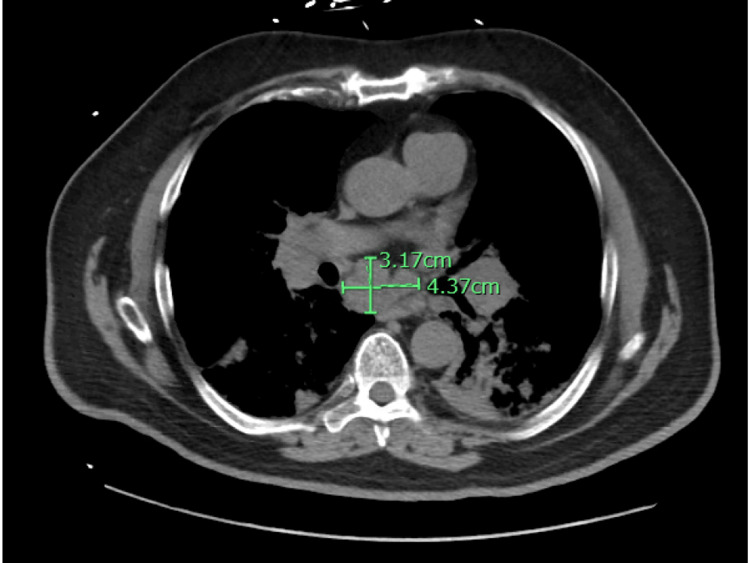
CT chest without contrast (axial view) showed extensive mediastinal and hilar adenopathy with conglomerate subcarinal lymph nodes measuring 4.37 x 3.17 cm.
